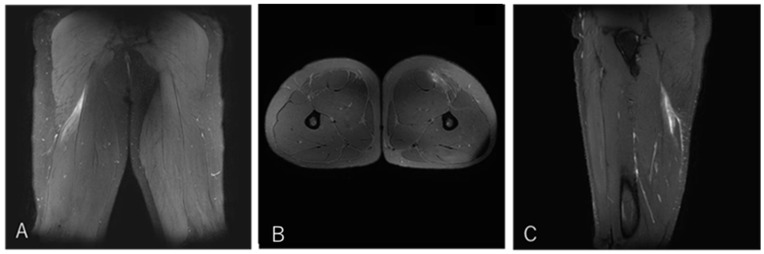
Case 4 MRI images: BFLH proximal MTJ injury, Type II. (A) (Coronal image): This is an injury to the proximal musculotendinous junction of the CT, with no evidence of tendon tortuosity. Haemorrhage is present on the BFLH side. (B) (Axial image): prone position, A haematoma is noted on the BFLH side of the CT. (C) (Sagittal image): A haematoma is observed in the cross-section of the BFLH.
